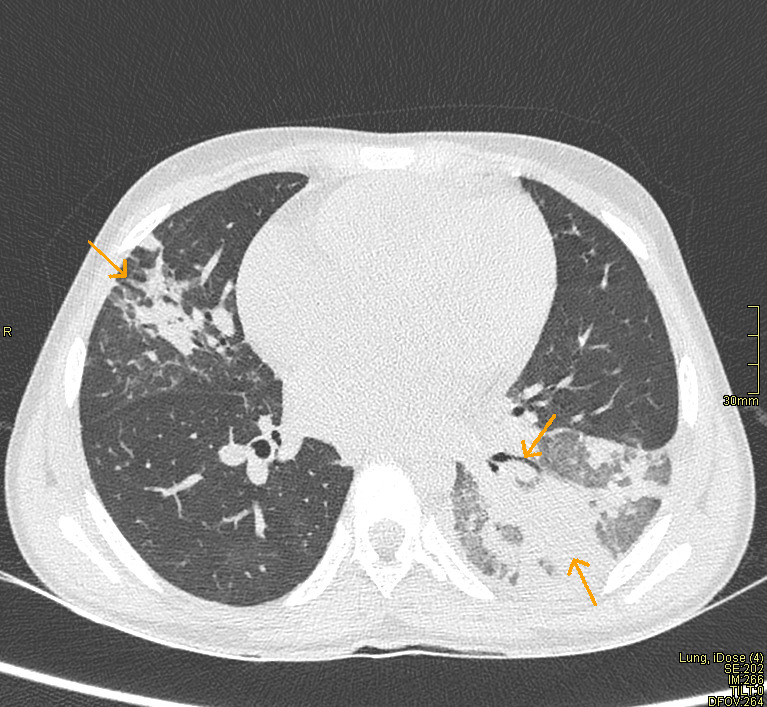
CT scan-axial image demonstrating left lower lobe consolidation (arrow on left-below) with air bronchogram (arrow on left-above) accompanied by peripheral sub-pleural patchy ground glass opacities (arrow right)
